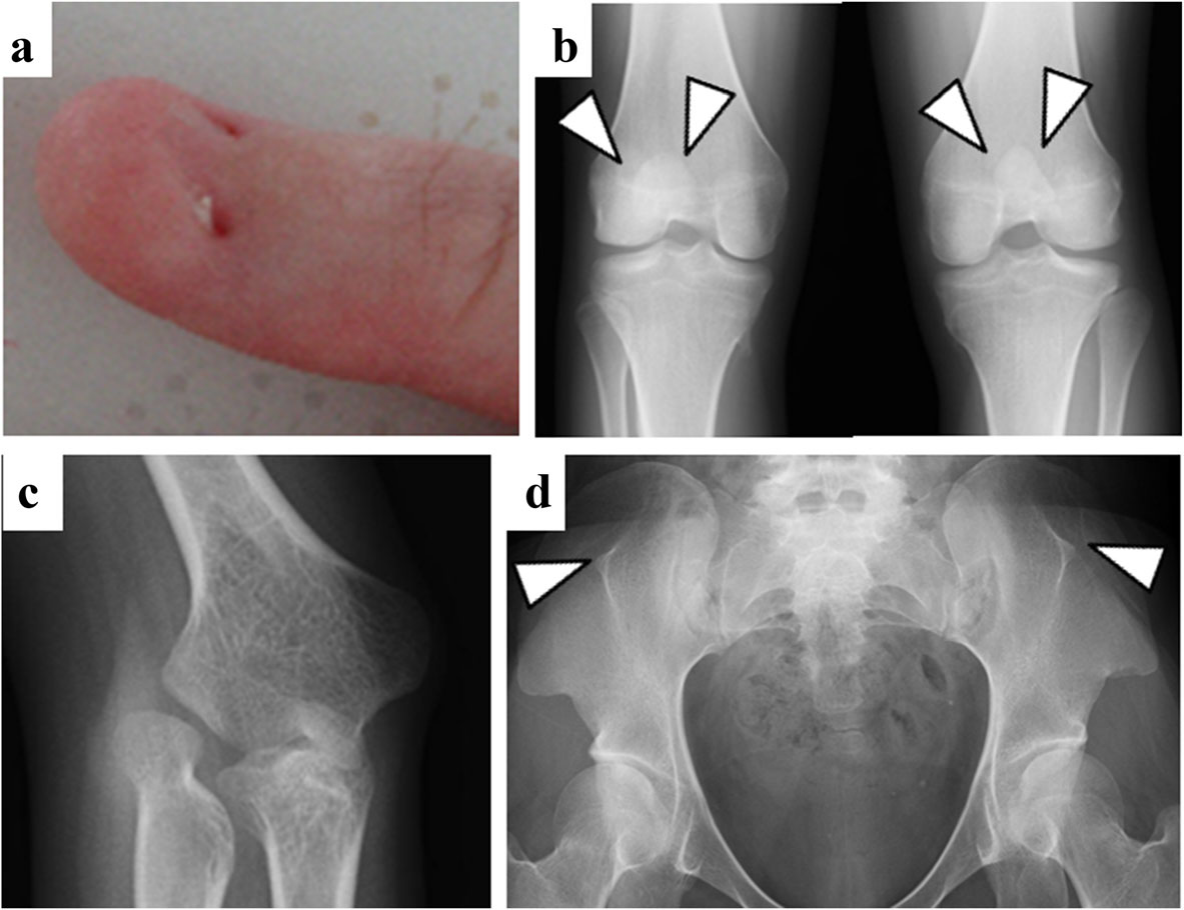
Nail abnormalities and orthopedic manifestations at the age of 24. She presented with poorly developed nails (a). An x-ray examination revealed hypoplastic patellas (b), dislocation of the elbows (c), and iliac horns in the pelvis (d)
